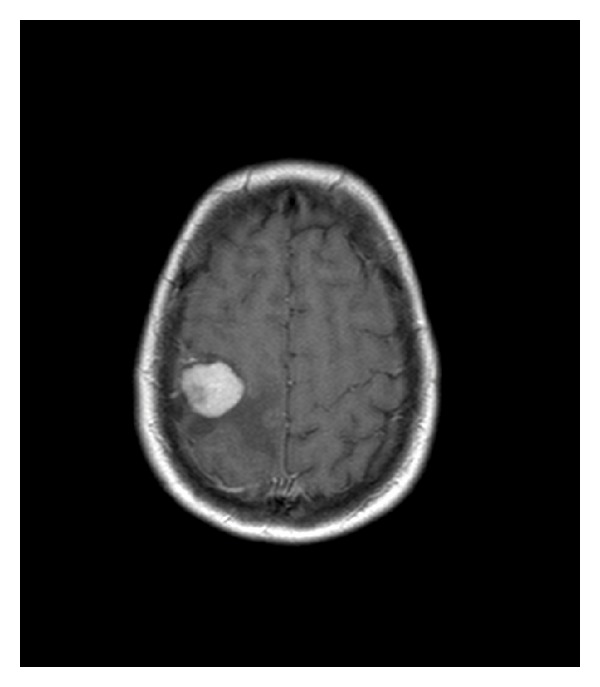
Preoperative MRI showing the mass in the right frontal lobe.
